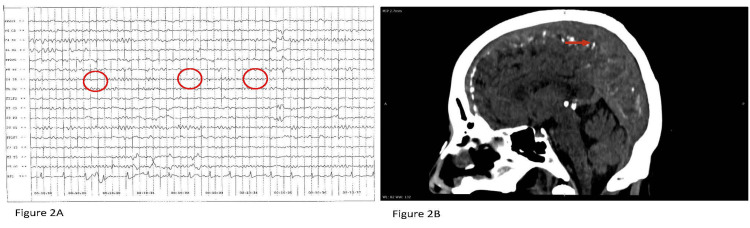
(A) EEG showing occasional right temporal slowing for Case 6 and (B) CT brain showing dural-based calcification for Case 6 EEG: electroencephalogram
